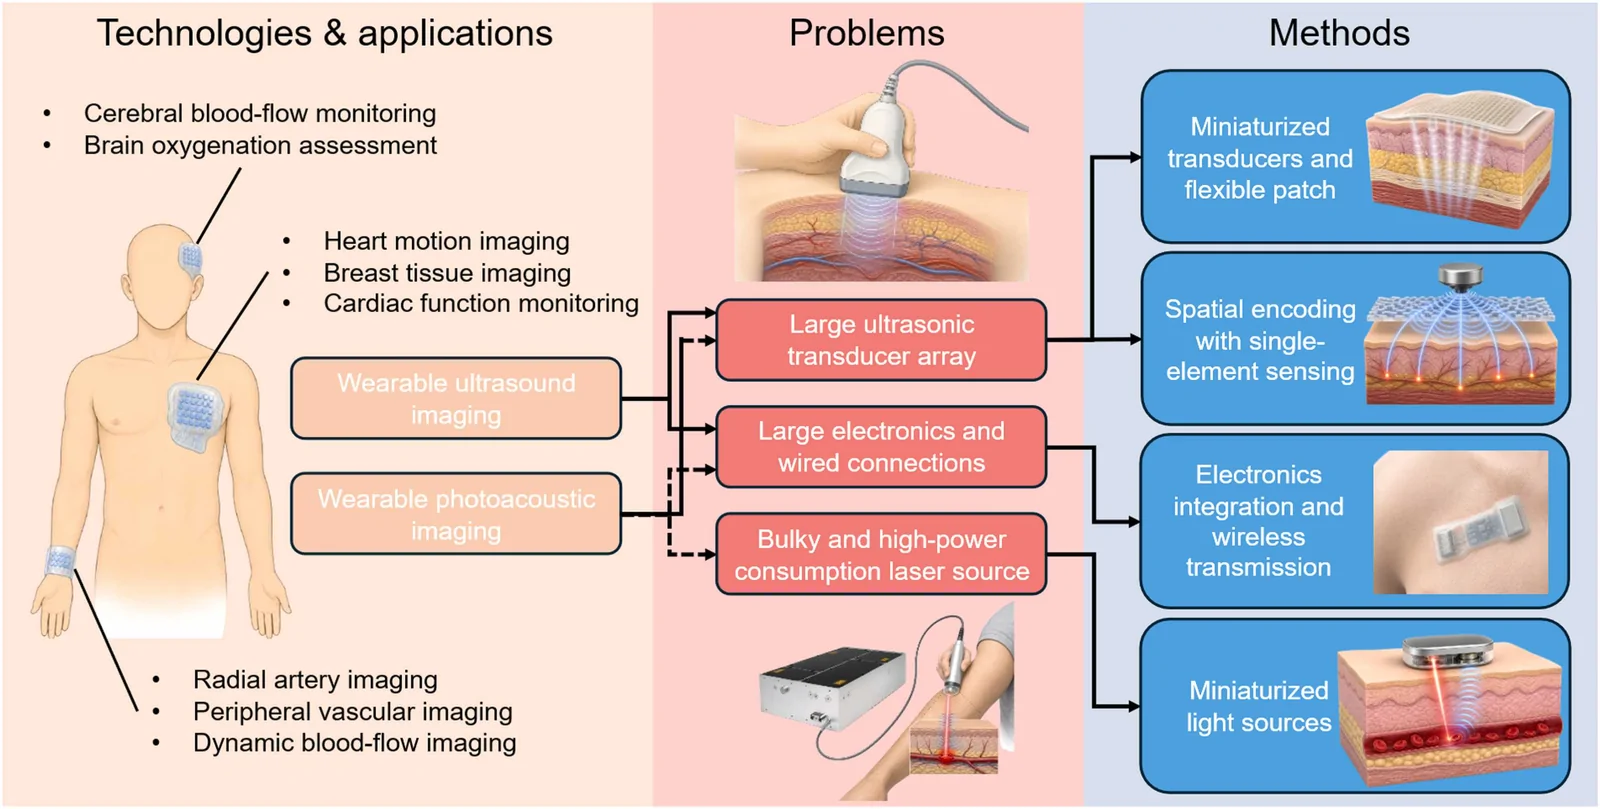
Overview of wearable ultrasound and photoacoustic imaging technologies.
